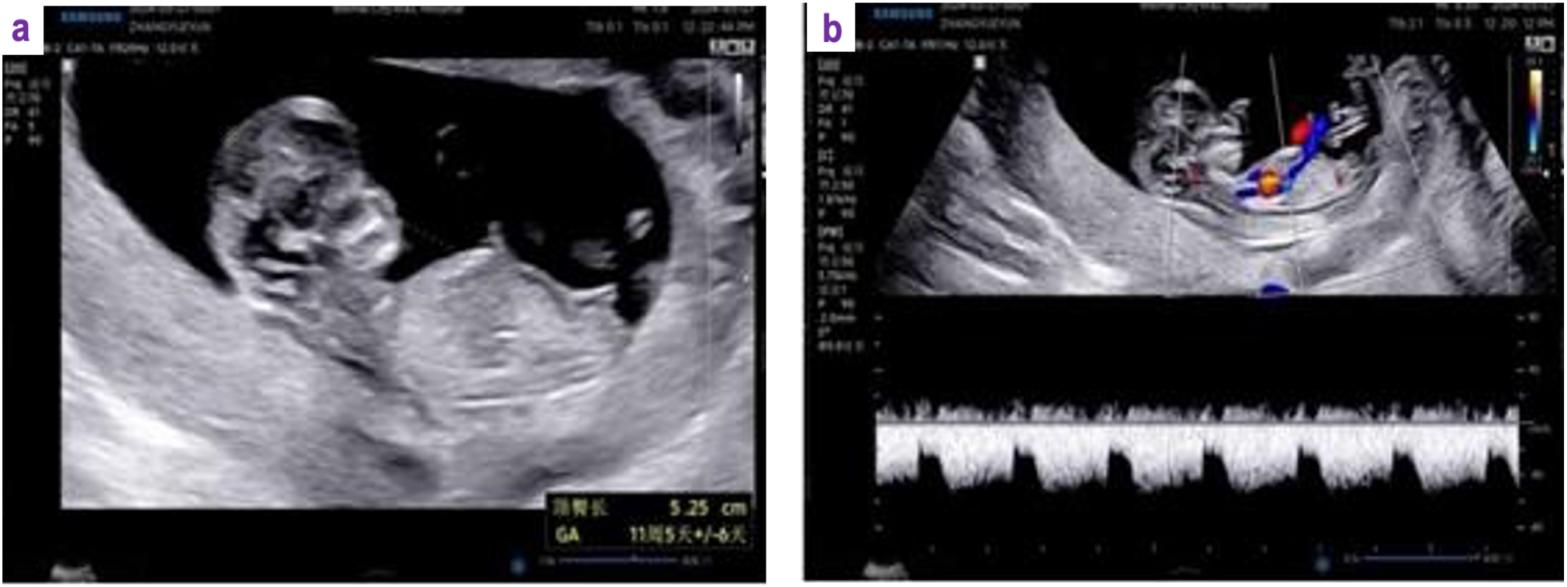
Ultrasound examination confirming successful pregnancy after fertility-sparing surgery. (a) A viable intrauterine pregnancy observed at 11$^{+}$4 weeks of gestation, with a crown–rump length of 52 mm and normal fetal cardiac activity. (b) Transvaginal ultrasound showing a cystic lesion in the right ovary measuring approximately 48 × 31 mm with homogeneous internal echoes.
